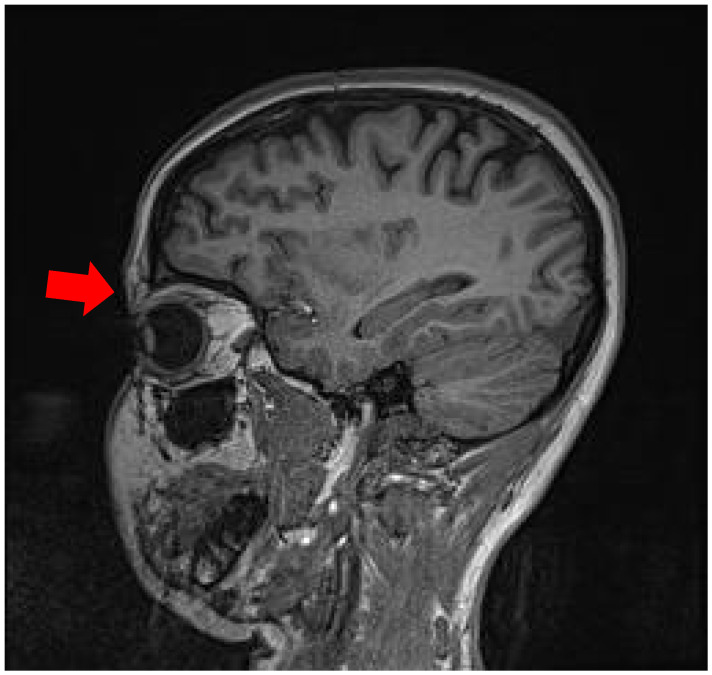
Orbital MRI: Bilateral lacrimal gland enlargement with homogeneous signal and periorbital fat stranding (arrow)—findings consistent with granulomatous dacryoadenitis.
